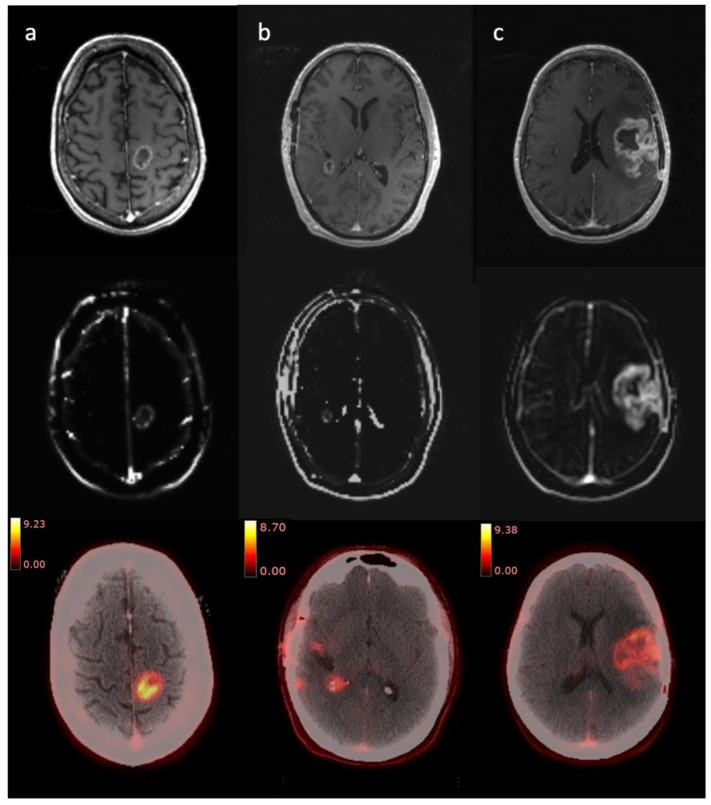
Selected Gd-T1 (top row), DCE-MRI (K^trans^, middle row) and PET-CT (bottom row, scale bar indicating SUV) images for three patients. (a) (left-hand column)—GBM 1, pre-radiotherapy; (b) (middle column)—GBM 3, pre-radiotherapy; (c) (right-hand column)—GBM 6, pre-radiotherapy.
